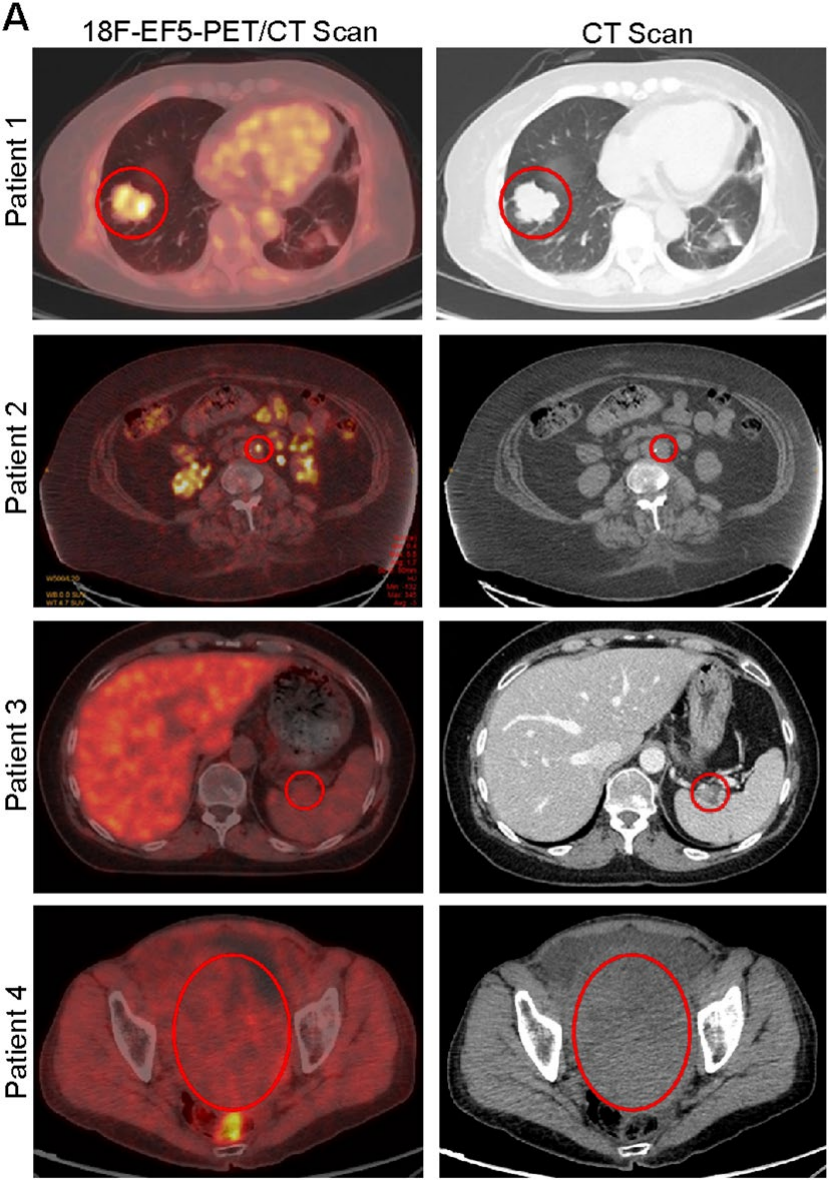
[^18^F]EF5-PET/CT positivity in tumors from CCOC patients. Representative images from each of the four CCOC patients. In two cases, patient 1 and patient 2, detectable EF5 positive was observed.
